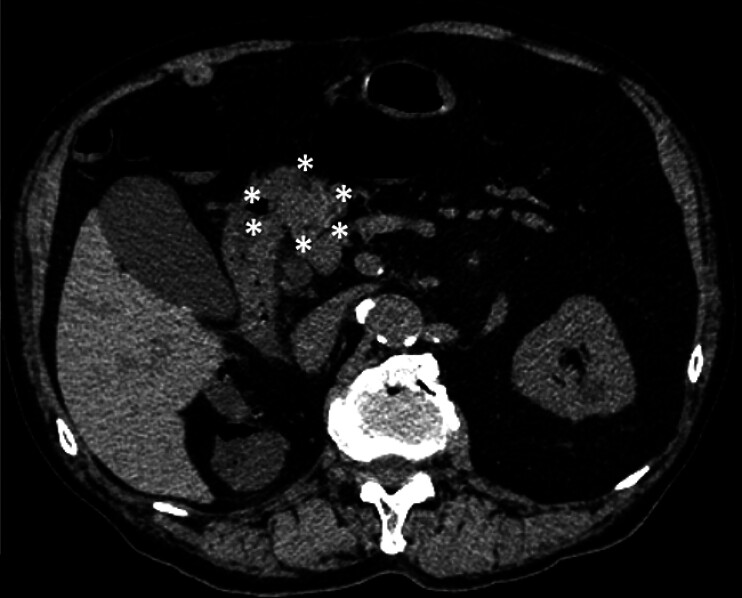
A screening non-contrast abdominal computed tomography (CT) scan revealed a suspicious 18-mm mass adjacent to the pancreatic head. The mass was hyperdense with well-defined borders, prompting further evaluation (asterisks).
